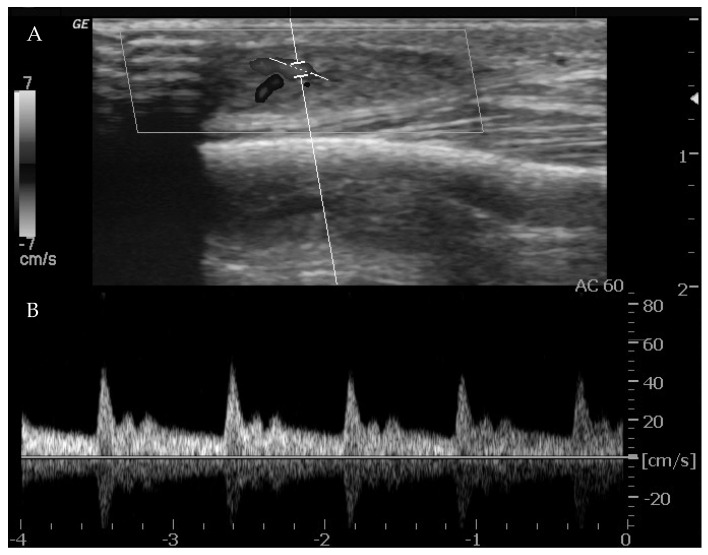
Ultrasound imaging with power Doppler on the thigh. (A). A well-defined, solid, ovoid lesion is seen in the subcutaneous plane, without calcification or cystic changes. (B). Significant flow is noted on color Doppler with an artery entering and exiting the lesion.
